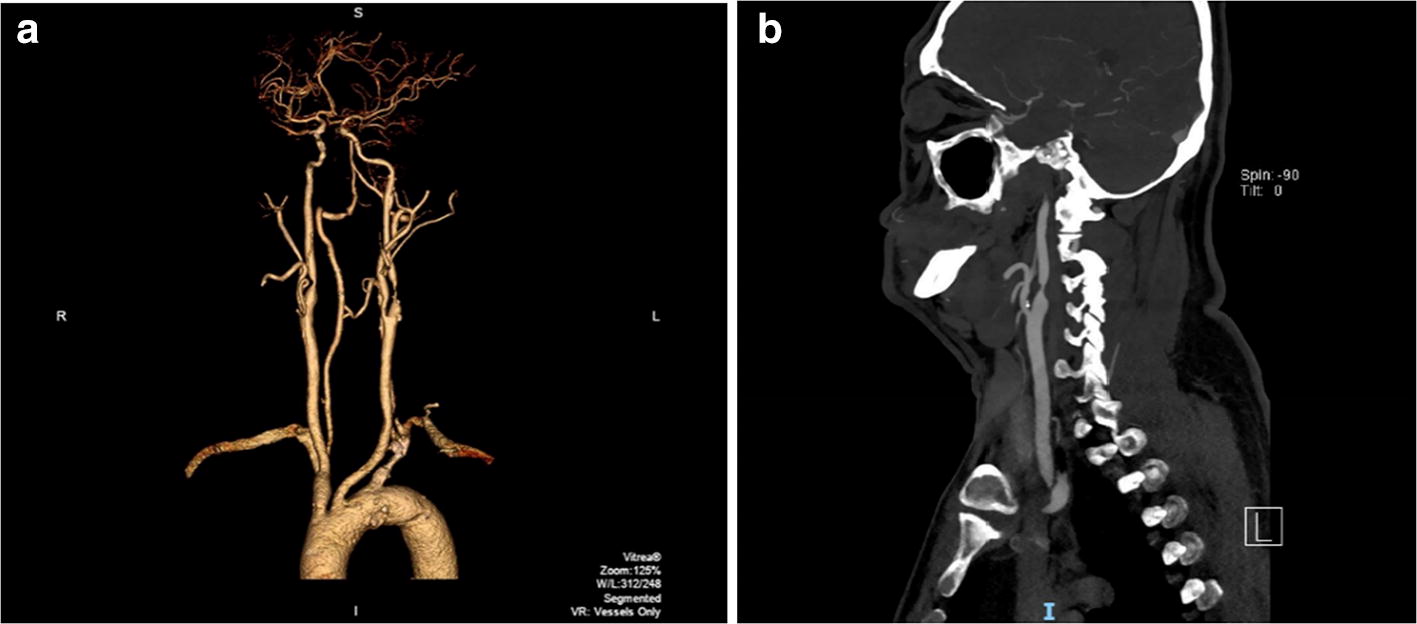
Carotid artery CTA: a 3D reconstruction, b 2D slice image (credits: National Heart Center Singapore)
